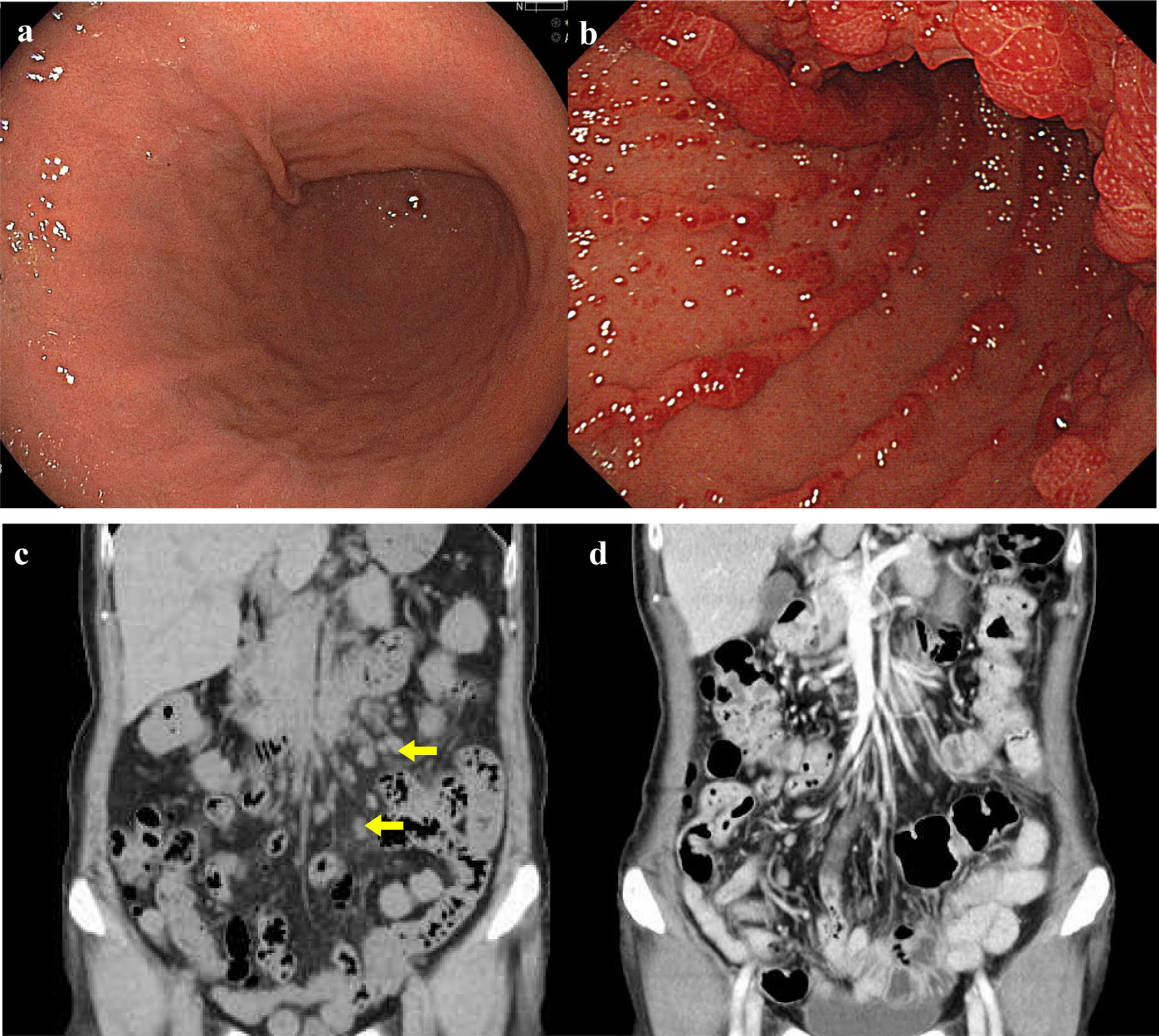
(a) Upper gastrointestinal endoscopy findings 1 year before visit. (b) Upper gastrointestinal endoscopy findings after consultation by referral. (c) CT scan of the abdomen at the time of referral showed multiple enlarged mesenteric lymph nodes. (d) A month after treatment, the mesenteric lymph node swelling had disappeared.
